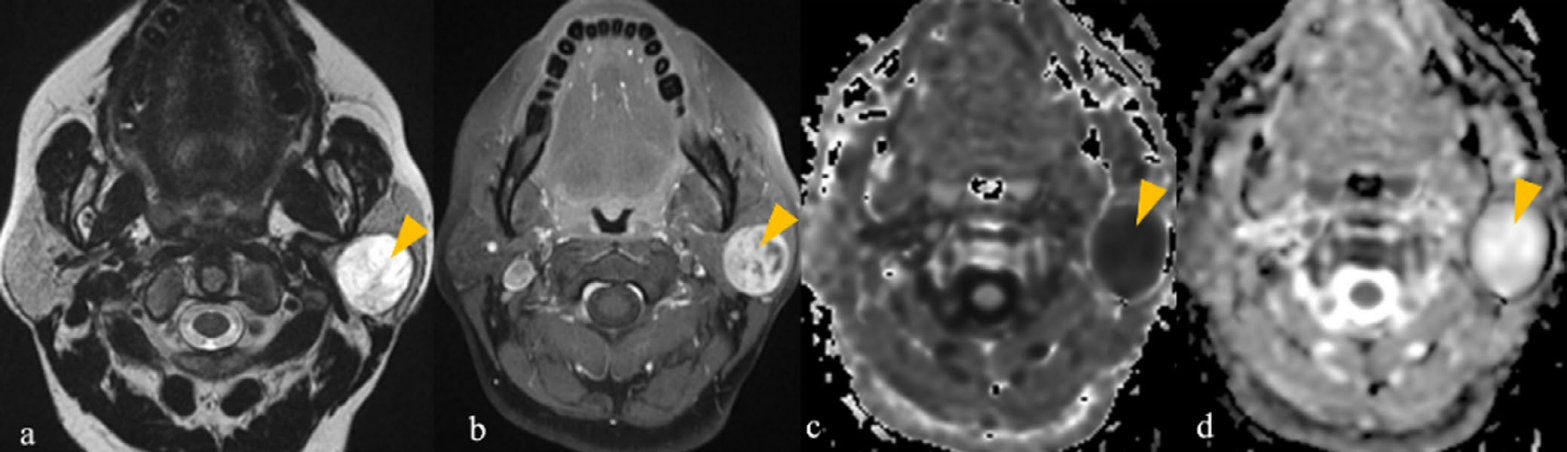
(a–d) 48‐year‐old female patient with Pleomorphic adenoma. (a) Axial T2WI shows a well‐defined encapsulated round‐shaped tumour in the superficial lobe of left parotid gland showing predominant hyperintense signal intensity (arrowhead). (b) Postcontrast T1WI shows heterogeneous enhancement (arrowhead). DWI (c) and ADC (d) show absence of diffusion restriction (arrowheads).
